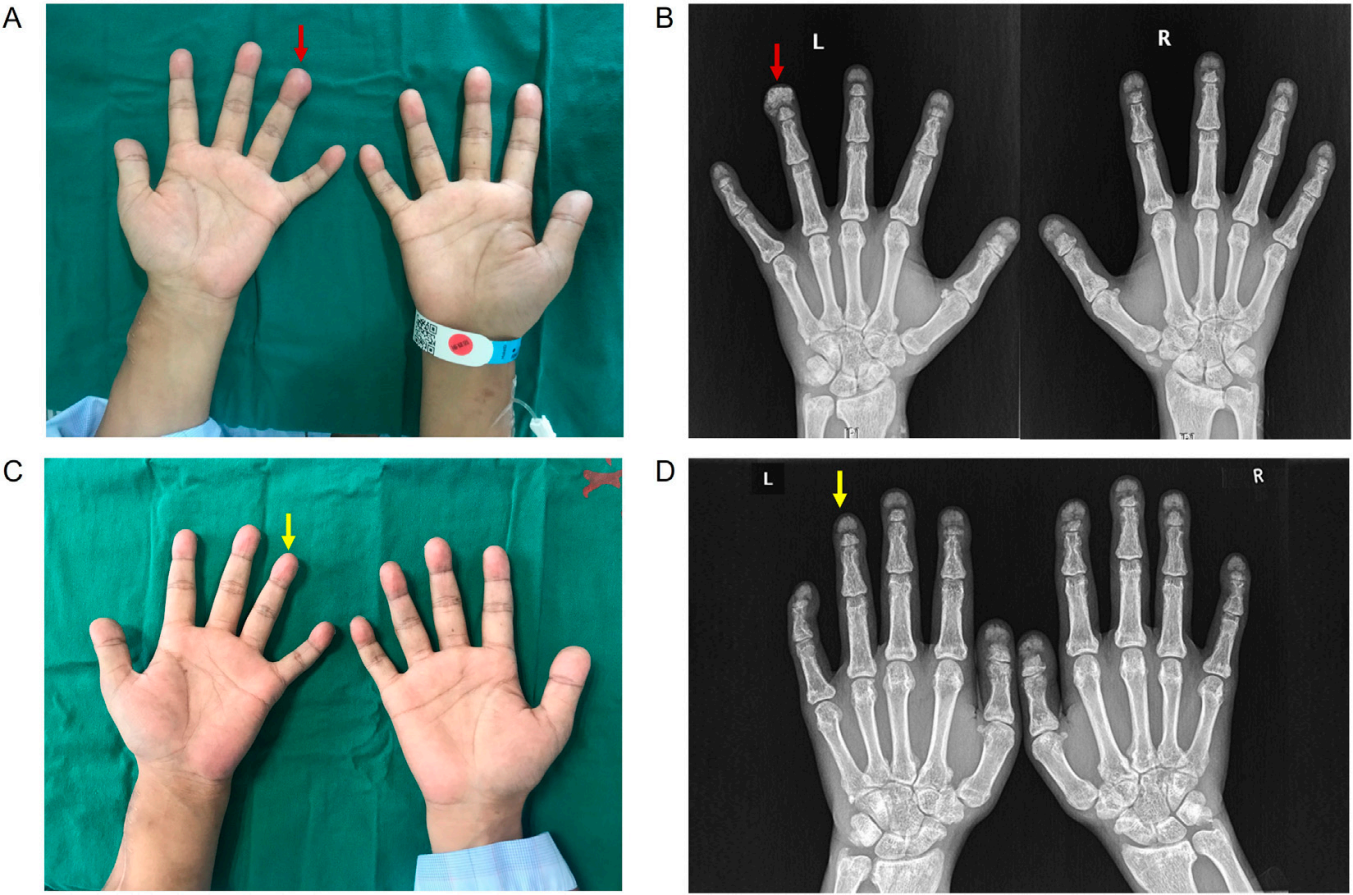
Clinical and imaging presentations of the patient. (A) The presence of subcutaneous nodule on left hand ring-finger. (B) X-rays of bilateral hands showed subcutaneous calcification on the extremity of left-hand ring-finger (red arrow). (C) Subcutaneous nodule on left hand ring-finger disappeared after STS treatment. (D) X-rays of bilateral hands showed the disappearance of subcutaneous calcification on the extremity of left-hand ring-finger after STS treatment (yellow arrow).
